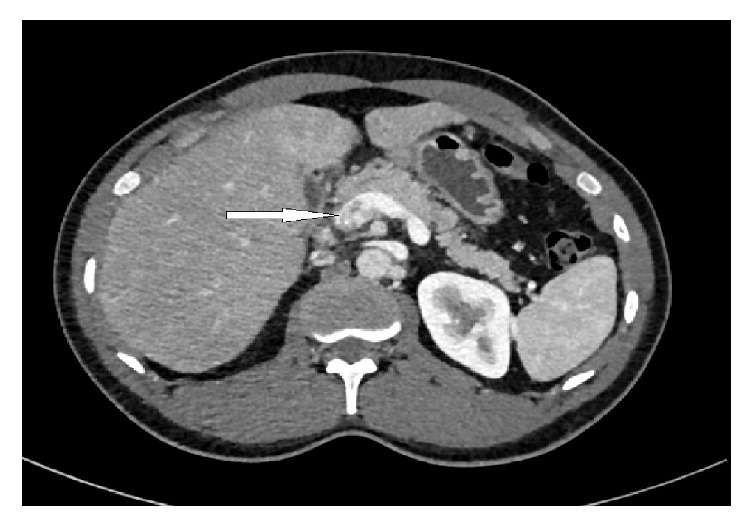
Contrast-enhanced CT scan abdomen showing partial filling defect in the portal vein (white arrow).
